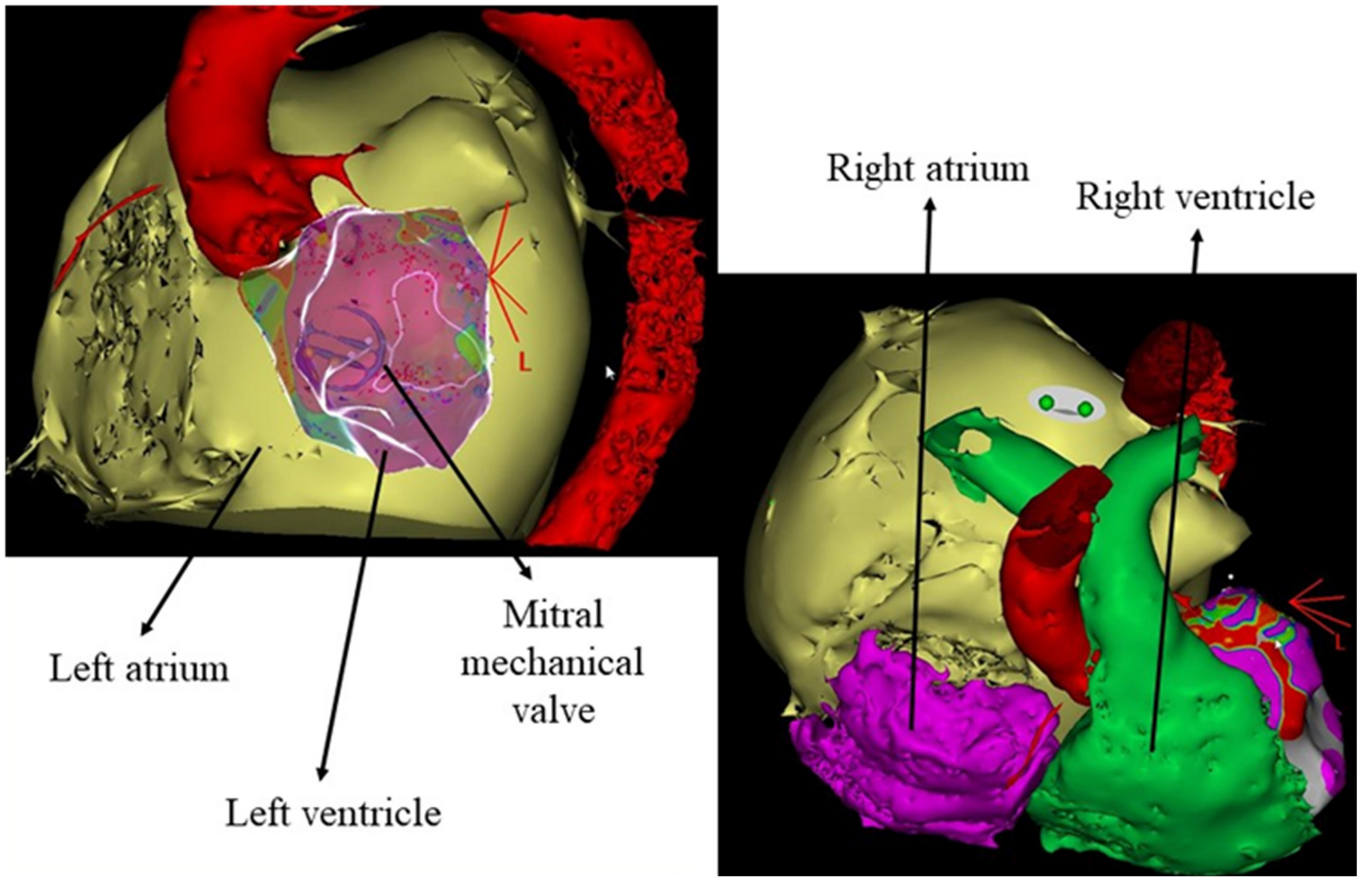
Anatomical details reconstructed from the preprocedural cardiac CT scan. Aorta in red, left atrium in yellow, right atrium in purple, and right ventricle in green.
